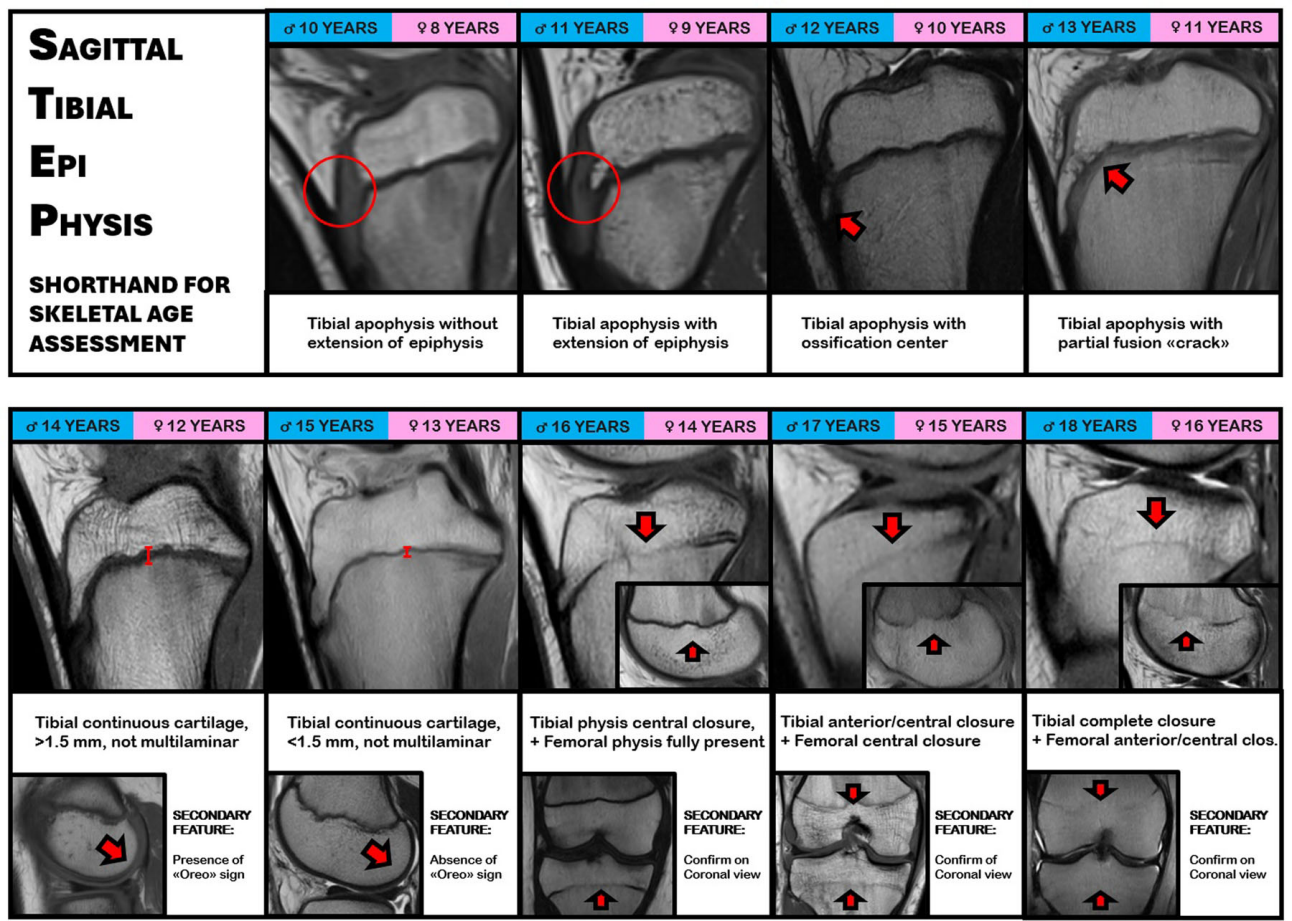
Sagittal tibial epi‐physis (STEP) shorthand for skeletal age assessment based on knee magnetic resonance imaging (MRI).
